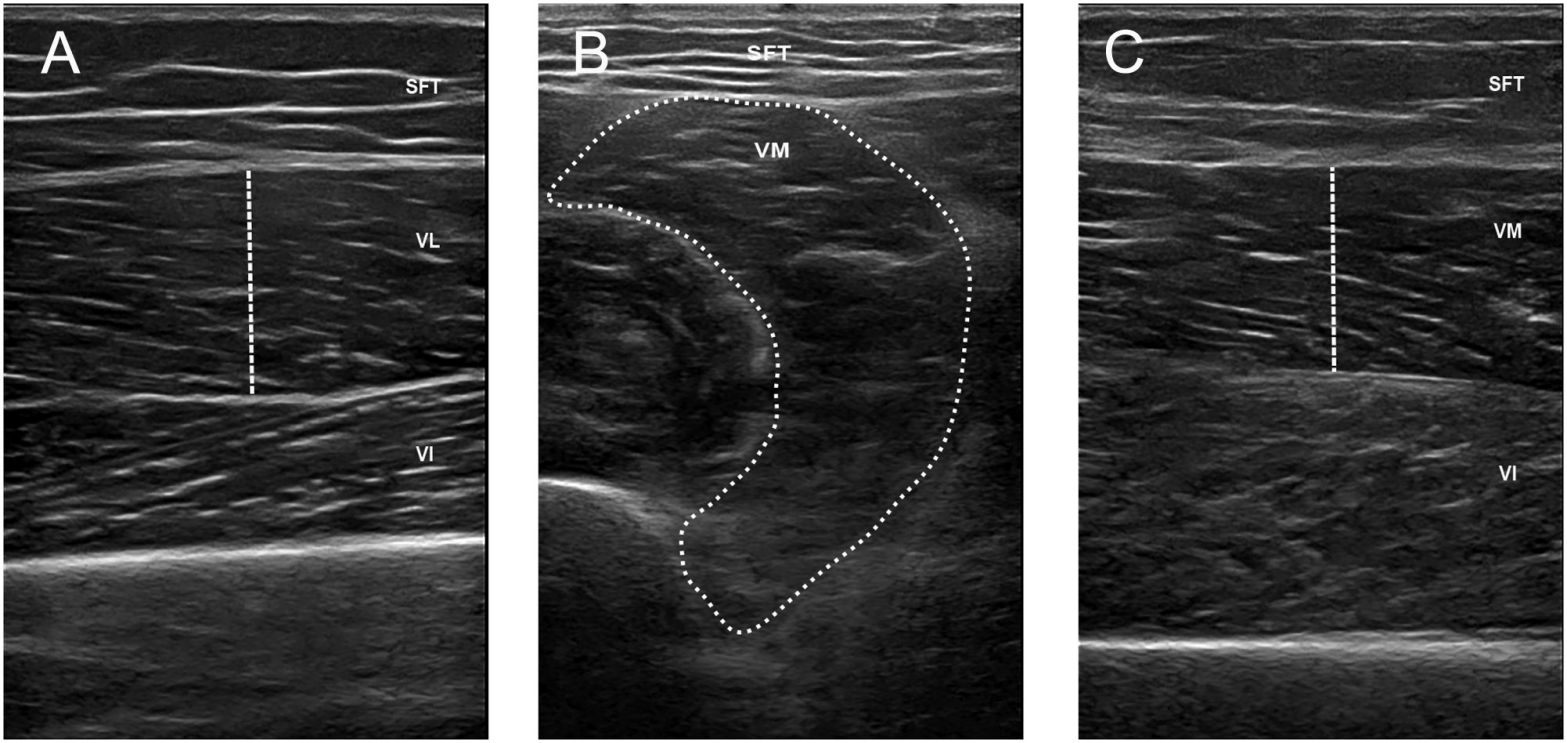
Examples of muscle ultrasound images in the HC group. A. Longitudinal section of the VL. B. Cross section of the VM. C. Longitudinal section of the VM. HC, healthy controls; SFT, subcutaneous fat thickness; VI, vastus intermedius; VL, vastus lateralis; VM, vastus medialis.
